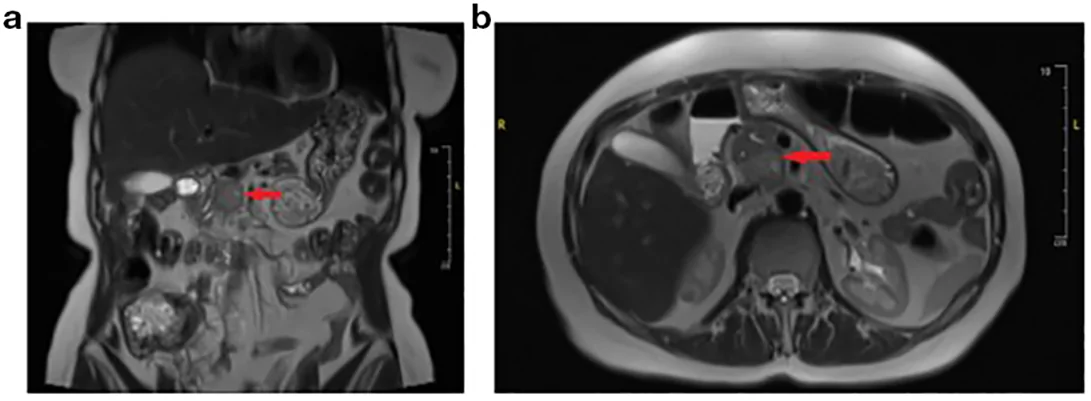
(a) T2 Abdomen MRI, coronal section. Pancreatic metastatic lesion of ovarian cancer (red headed arrow). (b) T2 Abdomen MRI, axial section. Pancreatic metastasizes (red headed arrow).
